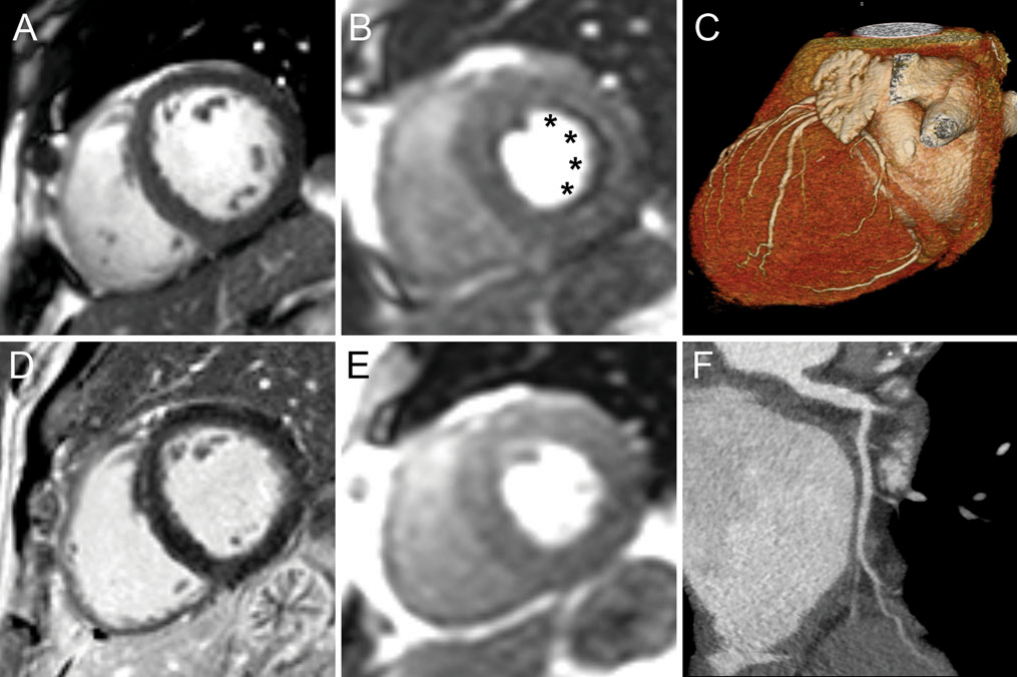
Example of a false positive CMR determined by prognosis in a 65 year old male with no prior cardiovascular history and intermediate pre-test probability for CAD and non-obstructive CAD on CTA. Short-axis cine CMR (a), subendocardial vasodilator stress induced perfusion defect involving the lateral and inferolateral walls (*asterisks*, b) which are not present on rest imaging (e). Delayed enhancement imaging is normal without evidence of myocardial infarction (f). Coronary CTA 3D surface (c) shows normal distribution of vessels to the lateral wall and non-obstructive CAD disease of the left main and left circumflex (f)
